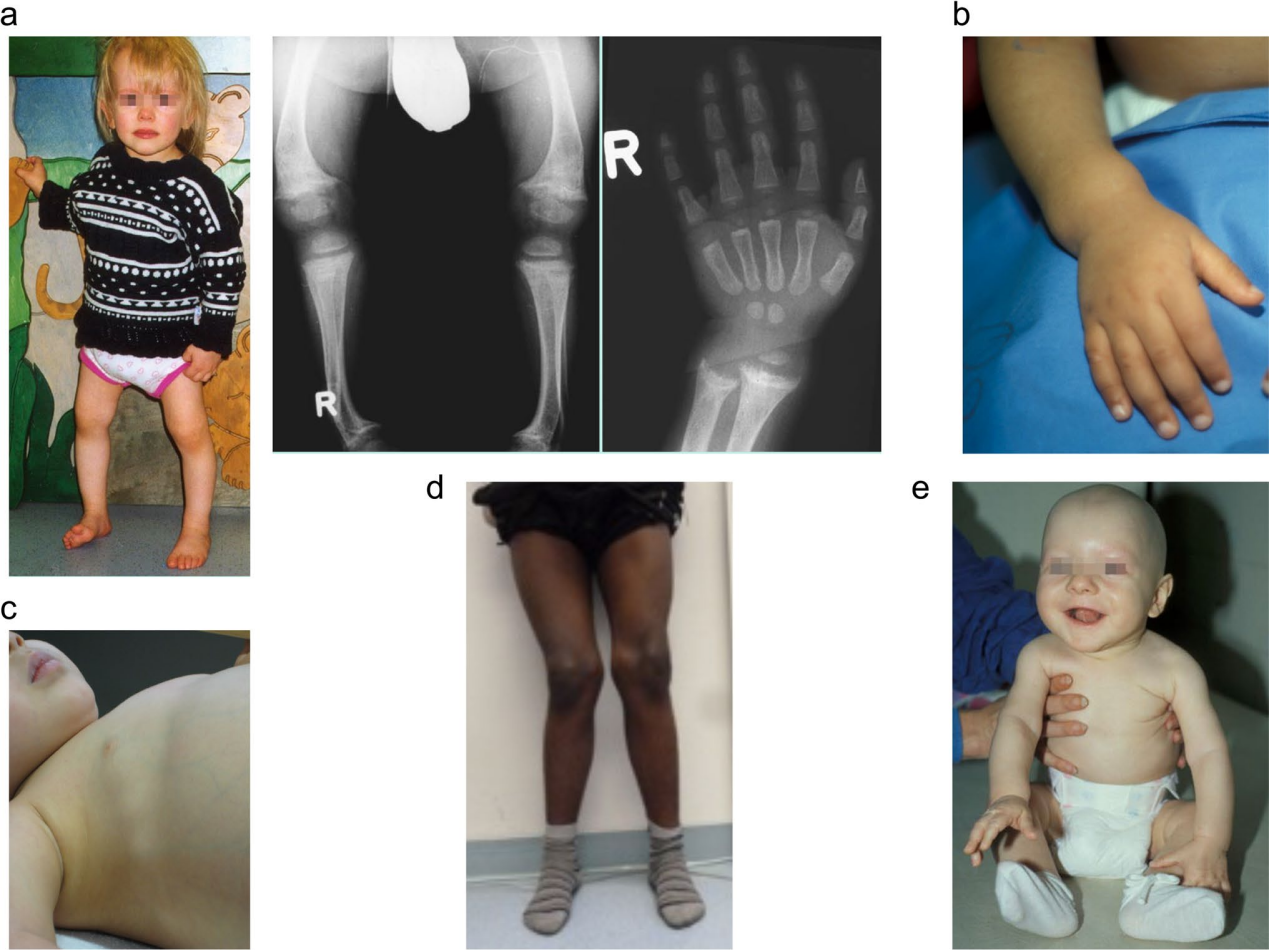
Clinical features of calcipenic rickets. (a) 18-month-old girl presenting with genu vara, and widening of the growth plates and metaphyseal fraying on X-rays, caused by nutritional rickets. (b, c) Two infants with widening of the wrist and rachitic rosary, respectively, due to nutritional rickets. (d) 14-year-old boy with genu valga due to nutritional rickets. (e) Infant with alopecia due to vitamin D-dependent rickets type 2A. Figure 2a, b, and e are reproduced from Schnabel and Haffner with permission [109]
